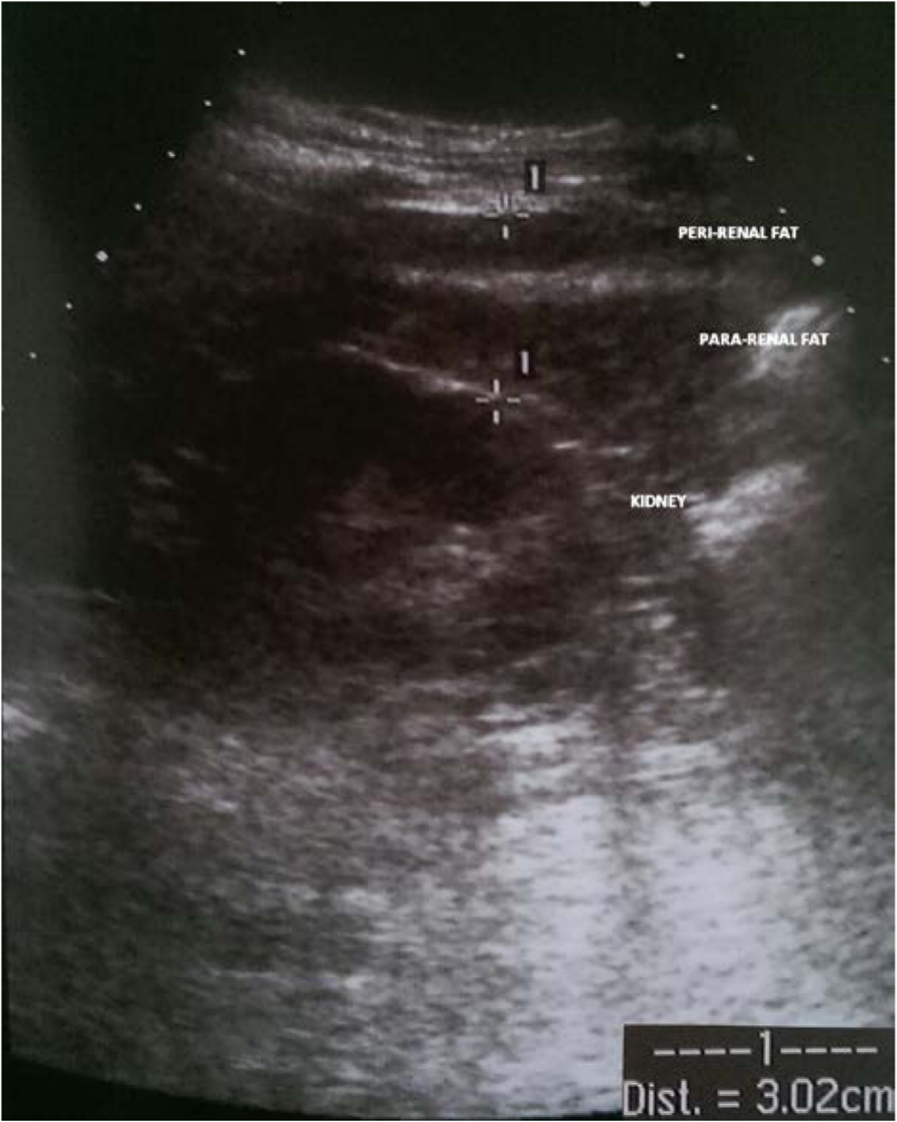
PUFT = para- and perirenal ultrasonographic fat thickness
